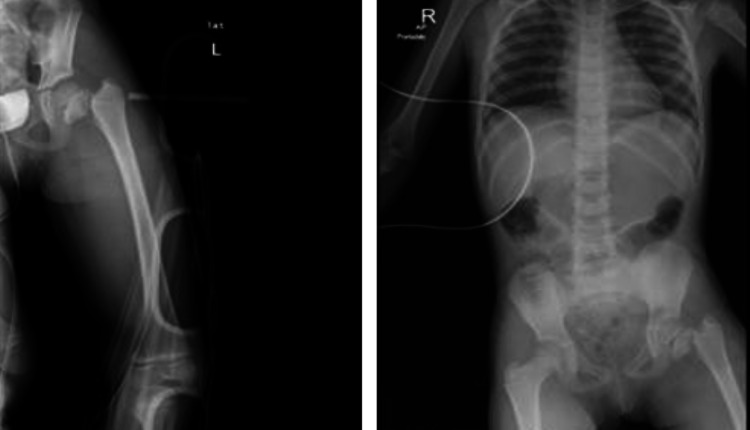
X-ray of left hip and pelvis
